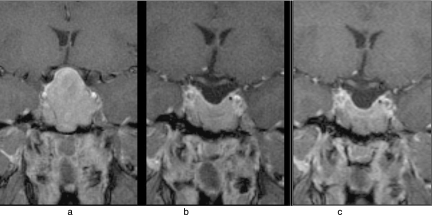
Coronal MR images in a patient with macroadenoma (a) at baseline (volume 22 702 mm^3^), (b) after 24 weeks (volume 15 285 mm^3^) and (c) after 48 weeks (volume 11 746 mm^3^) of treatment with octreotide LAR.
